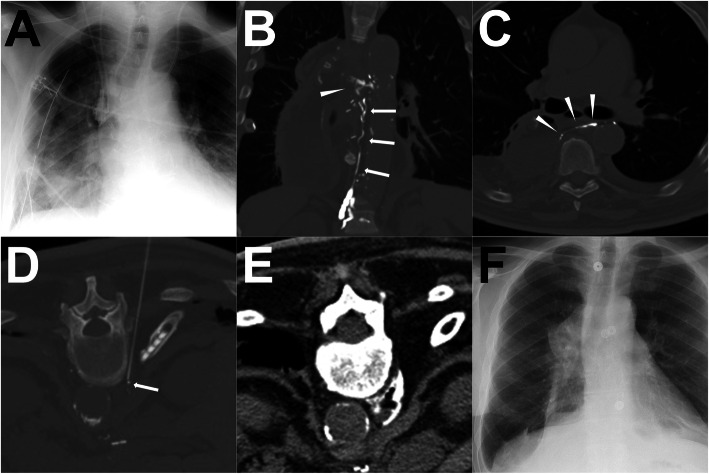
A patient underwent ALVS procedure after post-LAG CT. Note: After the surgery, the right chylothorax appeared with hyperdensity of the right lower lung in the chest radiogram (a). The coronal MIP images of post-LAG CT demonstrated a definite rupture (white arrowhead) of the thoracic duct (white arrows) and the extravasation to the right pleural cavity (b). The axial MIP image demonstrated a clear rupture of the thoracic duct (c). The lower thoracic duct was regarded as the target afferent LV for the ALVS and a 21-G Chiba needle was used to puncture close to the lower thoracic duct (d). Afterwards, 1 ml contrast followed with 4.0 ml 95% Ethanol being injected. The post-ALVS CT scan showed an ideal distribution of the sclerosant around the target thoracic duct (e). Eleven (11) days after ALVS, the chest radiogram shows no obvious recurrence of the chylothorax (f). Abbreviations: ALVS – afferent lymphatic vessel sclerotherapy; post-LAG CT – post-lymphangiographic computed tomography; MIP – maximum intensity projection; LV – lymphatic vessel
